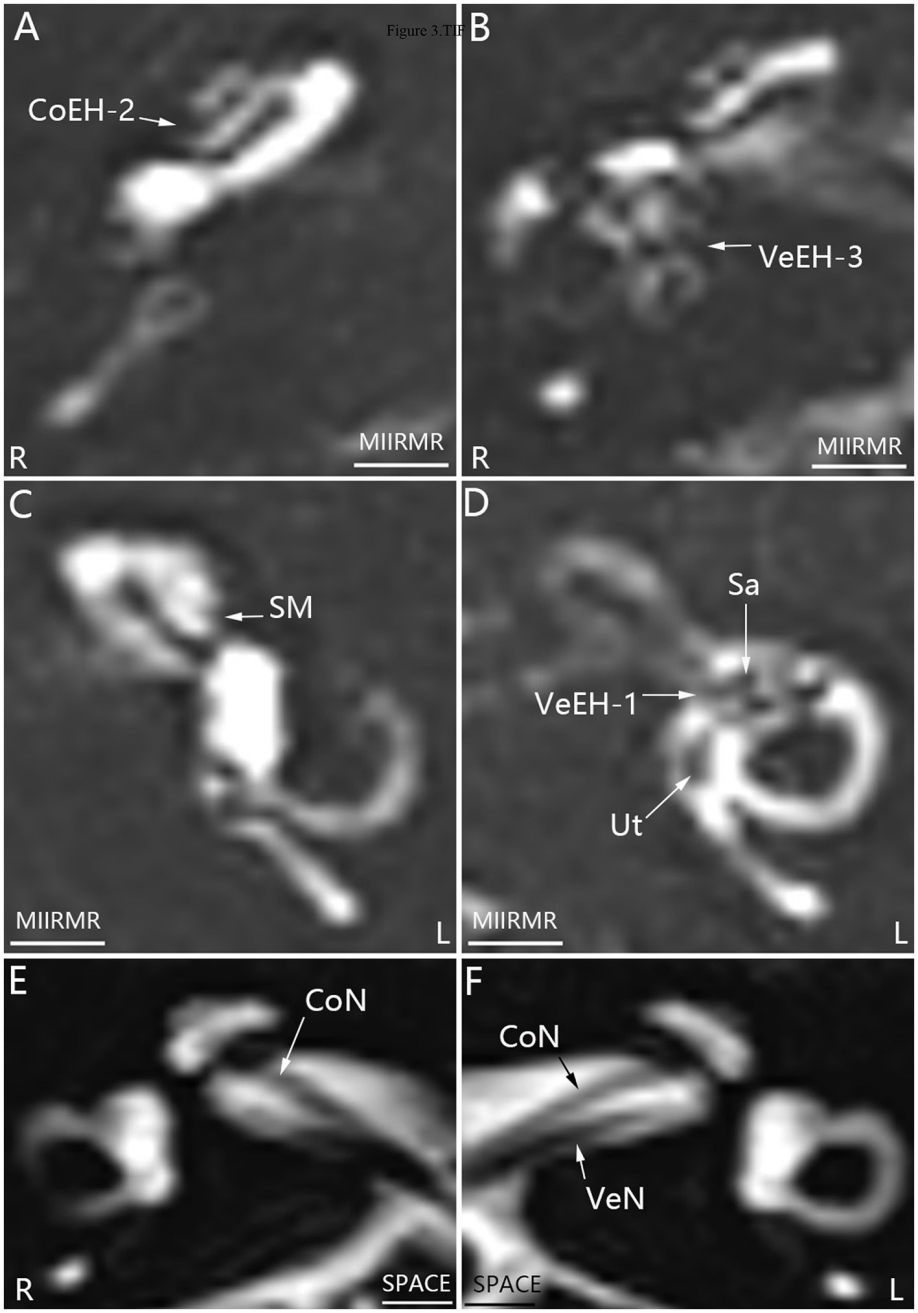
Representative MRI demonstrating EH in both ears in patient *E* with definite MD on the right side (A, B, D). MR images were taken using the MIIRMR sequence 24 h after intratympanic injection of 0.1 ml of 20-fold-diluted Gd-DTPA on both sides. There was no cochlear EH in the right ear (R) (C). Neither inner ear fibrosis nor vestibular schwannoma was found on the ipsilateral side imaged using the SPACE sequence (E, F). CoEH-2, grade 2 cochlear EH; CoN, cochlear nerve; L, left ear; Sa, saccule; Ut, utricle; VeEH-1 and VeEH-3, grade 1 and 3 vestibular EH; VeN, vestibular nerve. Scale bar = 3.0 mm.
